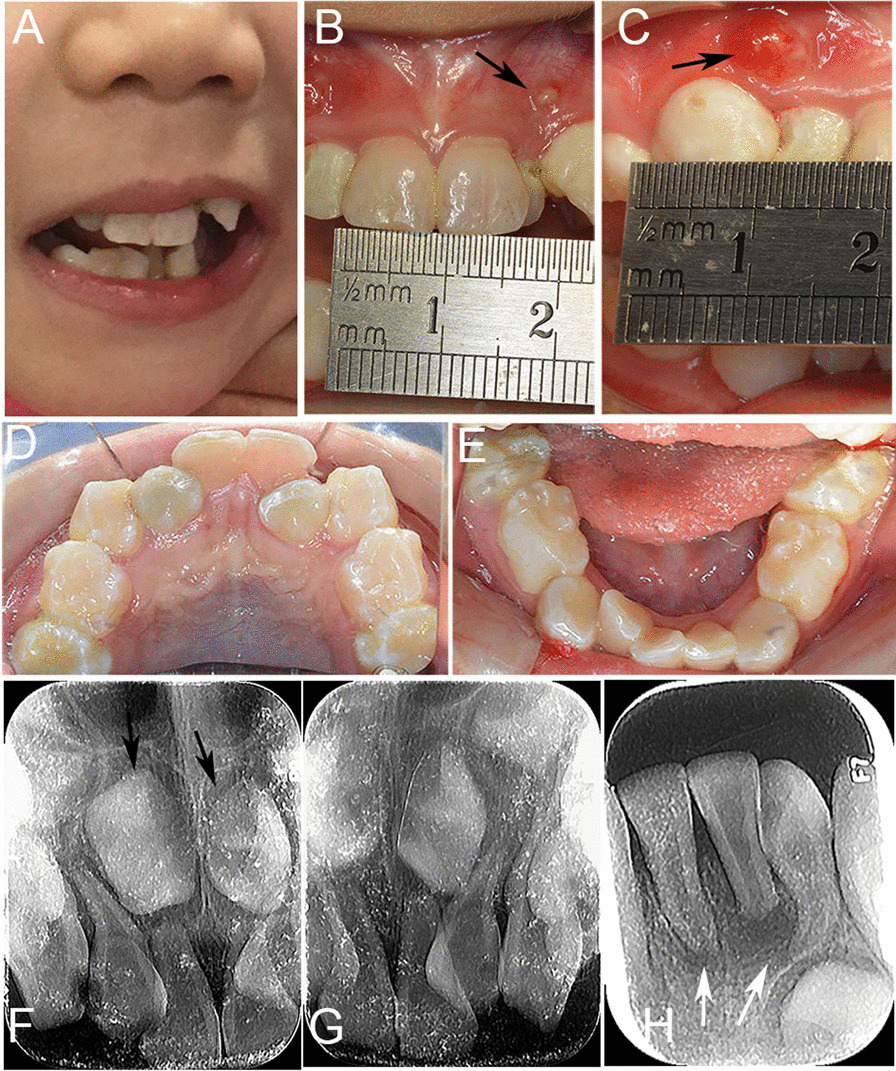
Clinical and radiographic features of case 1 with SAS. A The extraoral photo showing a flat philtrum, and a thin upper lip; B–E The intraoral photos showing macrodontia, crowded teeth, caries and periapical abscesses (black arrows); F–H The periapical radiographs of deciduous anterior teeth showing wide-open root apex and periapical low-density image (white arrows), and malformed and inverted permanent teeth 11 and 21 (black arrows), with the missing tooth buds of permanent teeth 31 and 41
